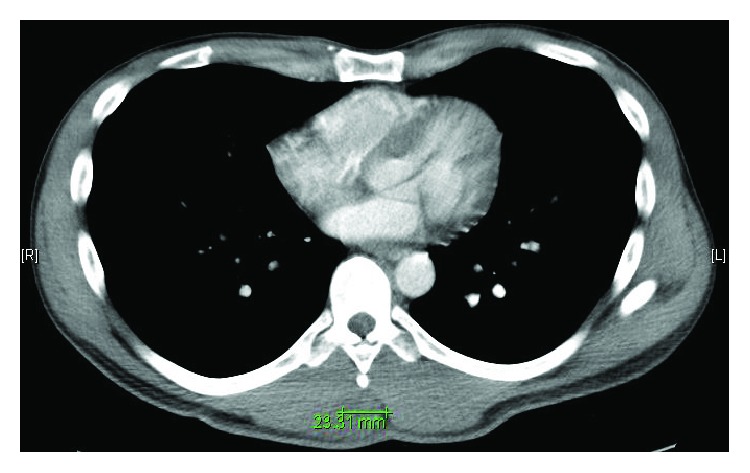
Mass noted on CT imaging.
